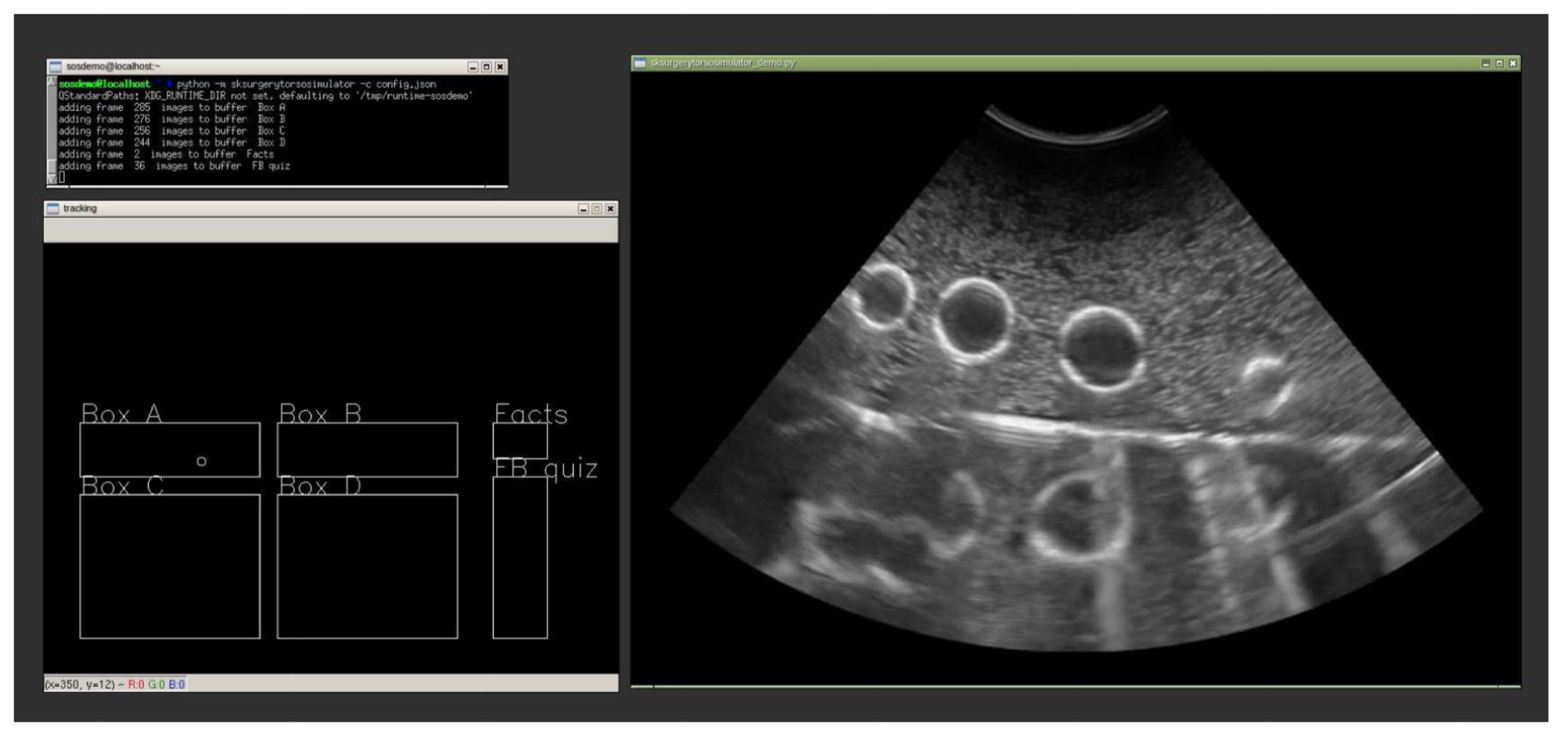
A screen shot of the system in use, top left is the command line and console output. Bottom left a window showing the tracker position with respect to the different parts of the video buffer. At right is the recorded ultrasound image. In this example the image is of a latex glove filled with water, which the user is trying identify by moving the probe around in “Box A”.
